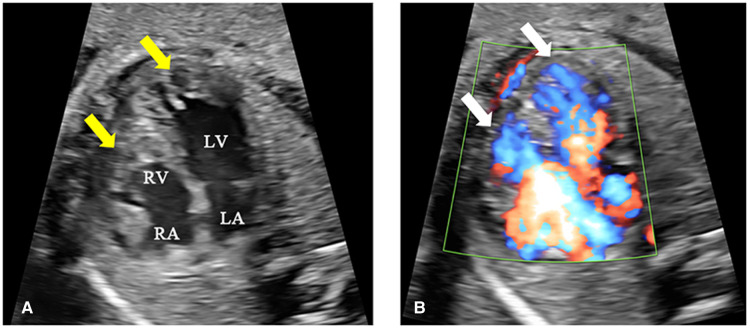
Fetal echocardiography at 25^+1^ weeks of gestation. (A) The two-dimensional ultrasound image shows increased numbers of prominent trabeculations and deep intertrabecular spaces in both ventricles (yellow arrow), especially at the left ventricular apex. The ratio of non-compaction (6 mm) to compaction (2 mm) was 3:1. (B) The color Doppler ultrasound image shows blood perfusing the intertrabecular recesses (white arrow). LA, left atrium; LV, left ventricle; RA, right atrium; RV, right ventricle.
